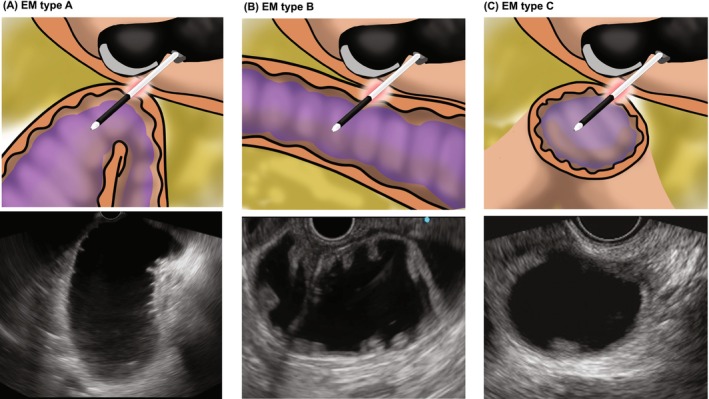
Endoscopic ultrasound (EUS)–based morphological classification (EM) of the targeted bowel loop. (A) Type A, the orientation of the target bowel lumen is along the longitudinal axis, which is perpendicular to the EUS probe. (B) Type B, the orientation of the target bowel lumen is along the horizontal axis, which is parallel to the EUS probe. (C) Type C, the target bowel lumen is seen in cross‐section as a circle.
